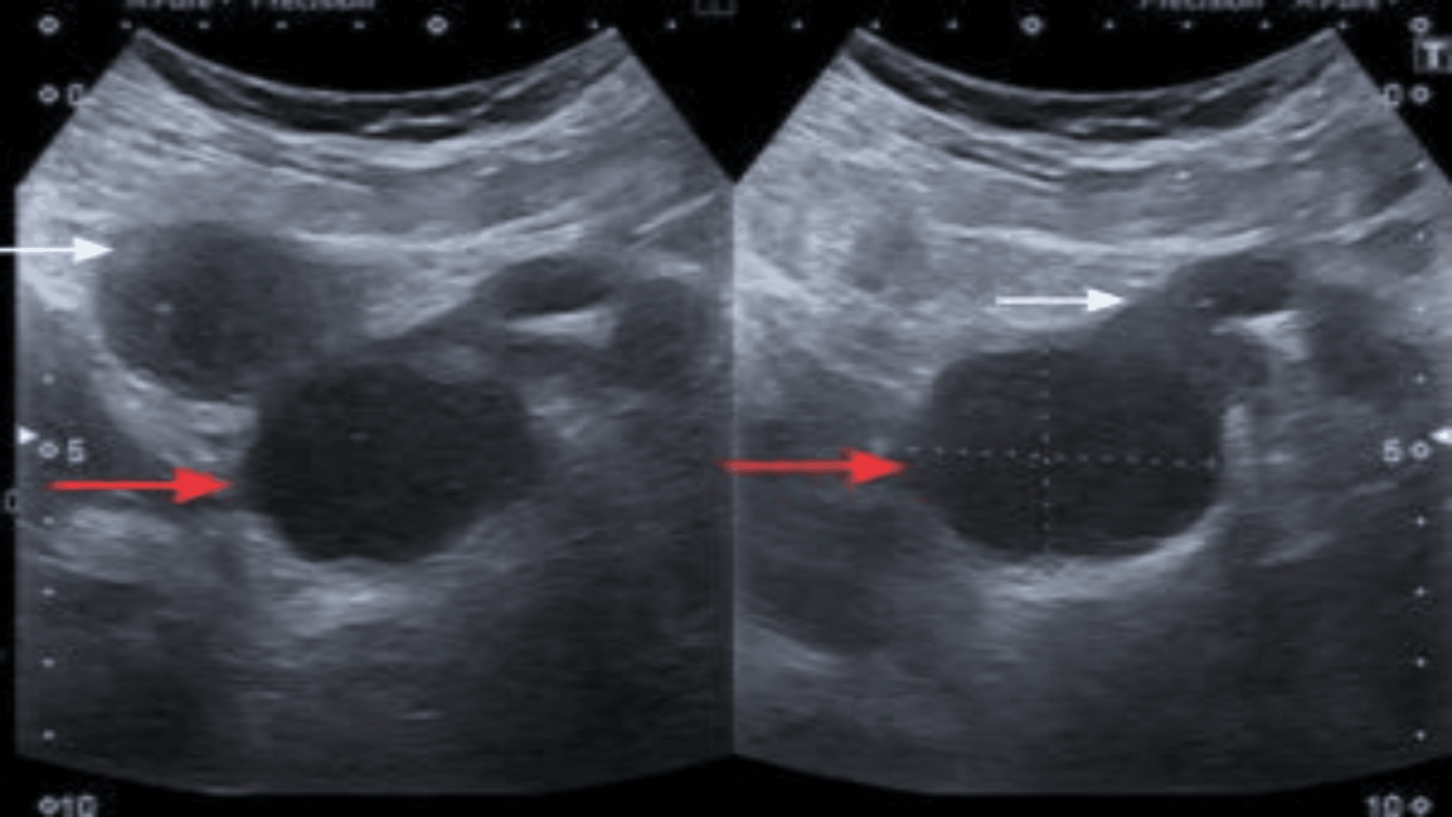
Transabdominal grayscale ultrasound demonstrating a large, complex left adnexal mass (cystic component indicated by red arrow and solid component indicated by white arrow). The lesion is predominantly cystic with internal septations and contains a solid, hypoechoic component. The findings are suspicious for malignancy and prompted further evaluation with MRI.
